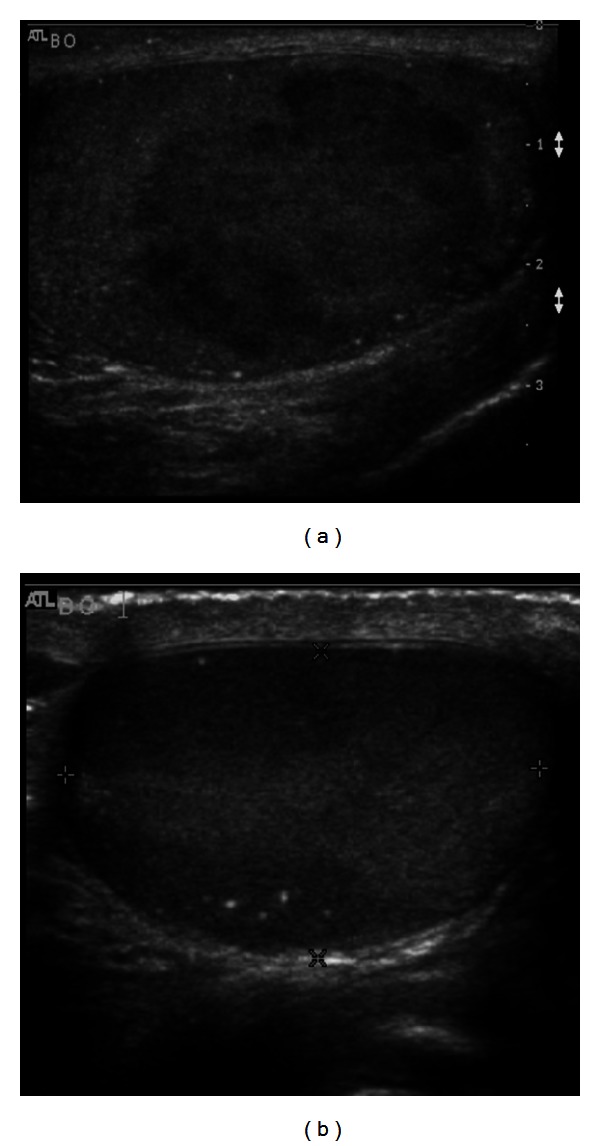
Scrotal ultrasound. (a) (R), (b) (L).
